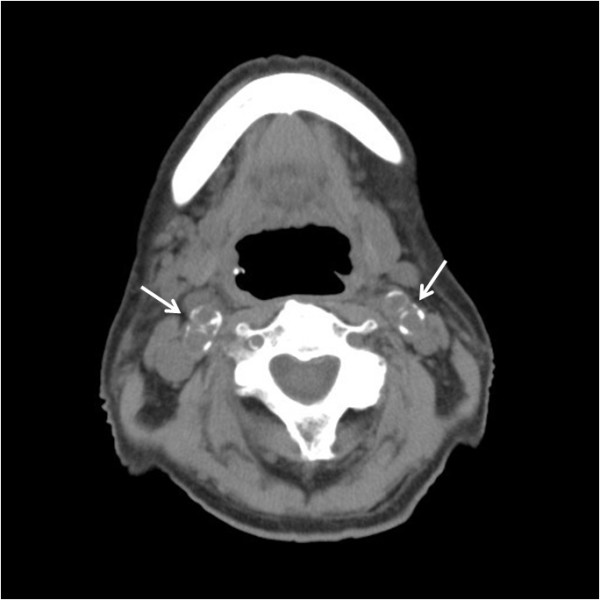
Carotid artery calcification detected by multi-detector computed tomography was seen at the origin of the bilateral internal and external carotid arteries (arrows).
